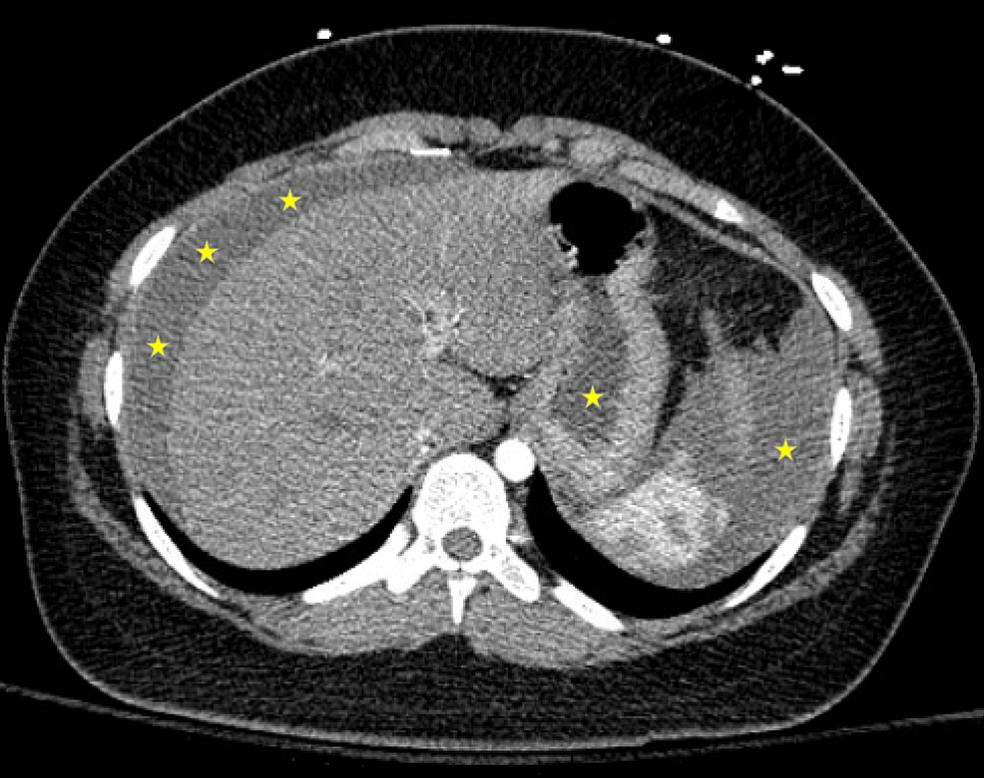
Non-contrast computed tomography (CT) scan demonstrating free fluid (asterisks).
